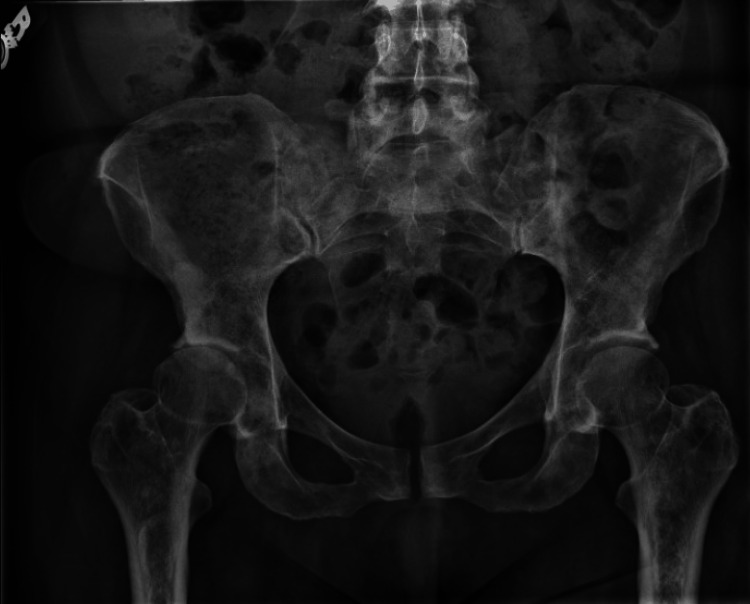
Pelvic radiograph demonstrating sclerotic osseous metastases (March 2023).
